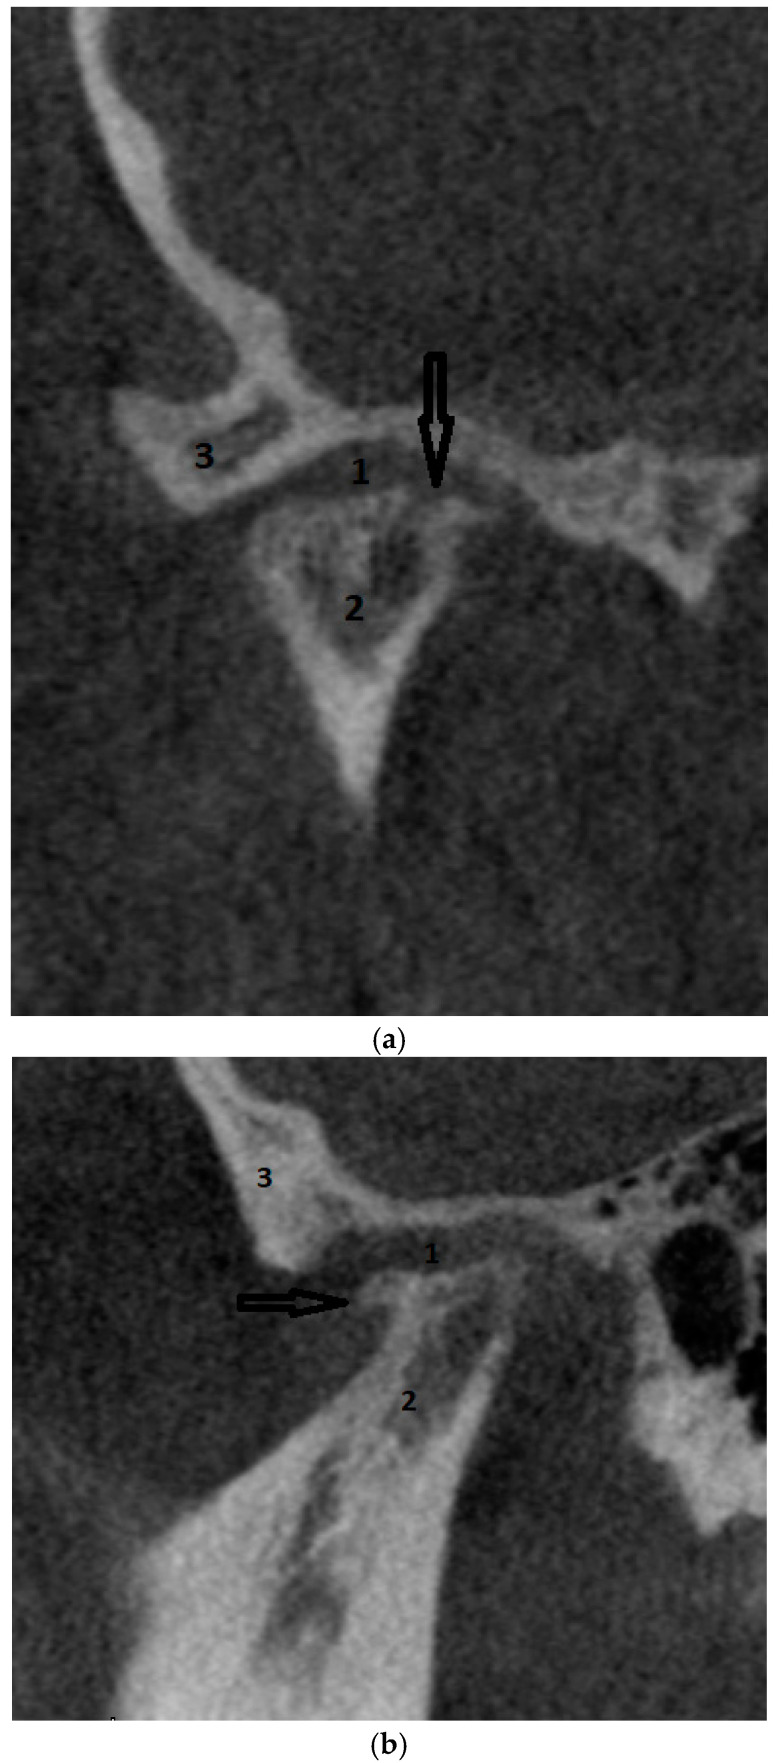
(a) CBCT (coronal view). Black arrow points to erosion and subchondral cyst. Anatomical structures of the TMJ: 1. Disc, 2. Condyle, 3. Temporal Bone; (b) CBCT (sagittal view). Black arrow indicates osteophyte. Anatomical structures of the TMJ: 1. Disc, 2. Condyle, 3. Temporal Bone.
